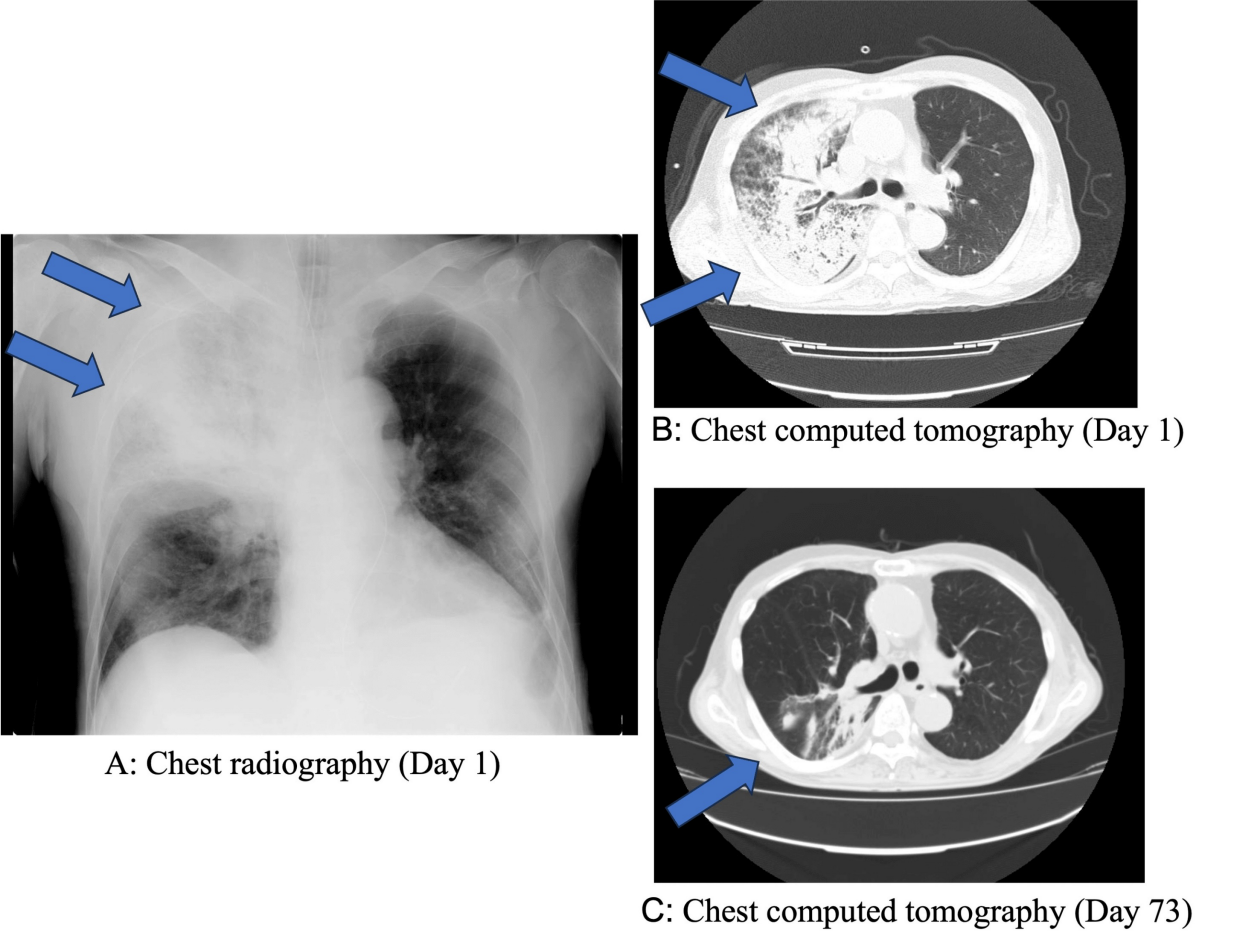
Chest radiograph and computed tomography (Case 1) Reticular shadow in the center of the right upper lung field (blue arrow).
